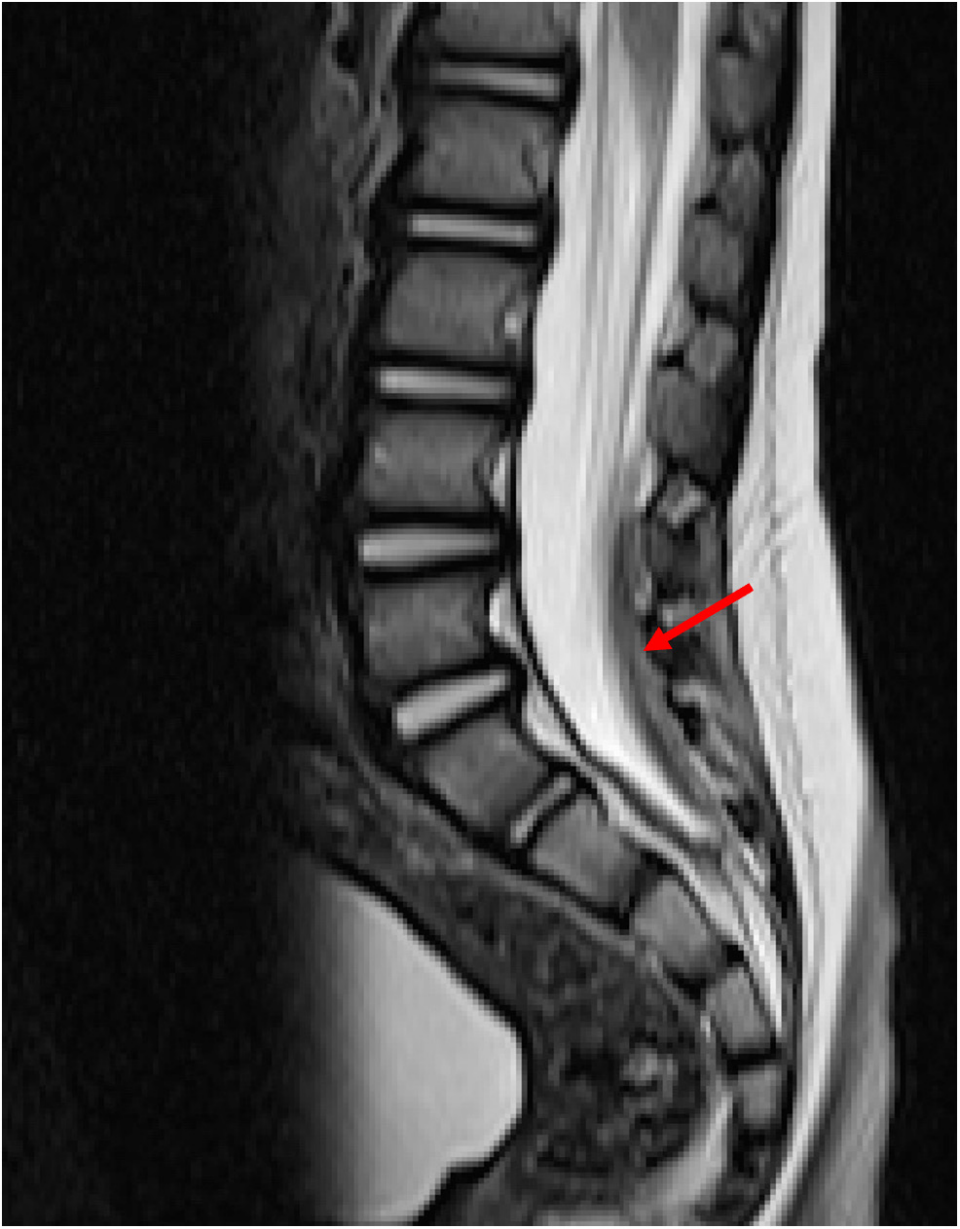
MRI spine 7 days after the fall shows a small subdural collection layering dependently in the lumbo‐sacral region.
